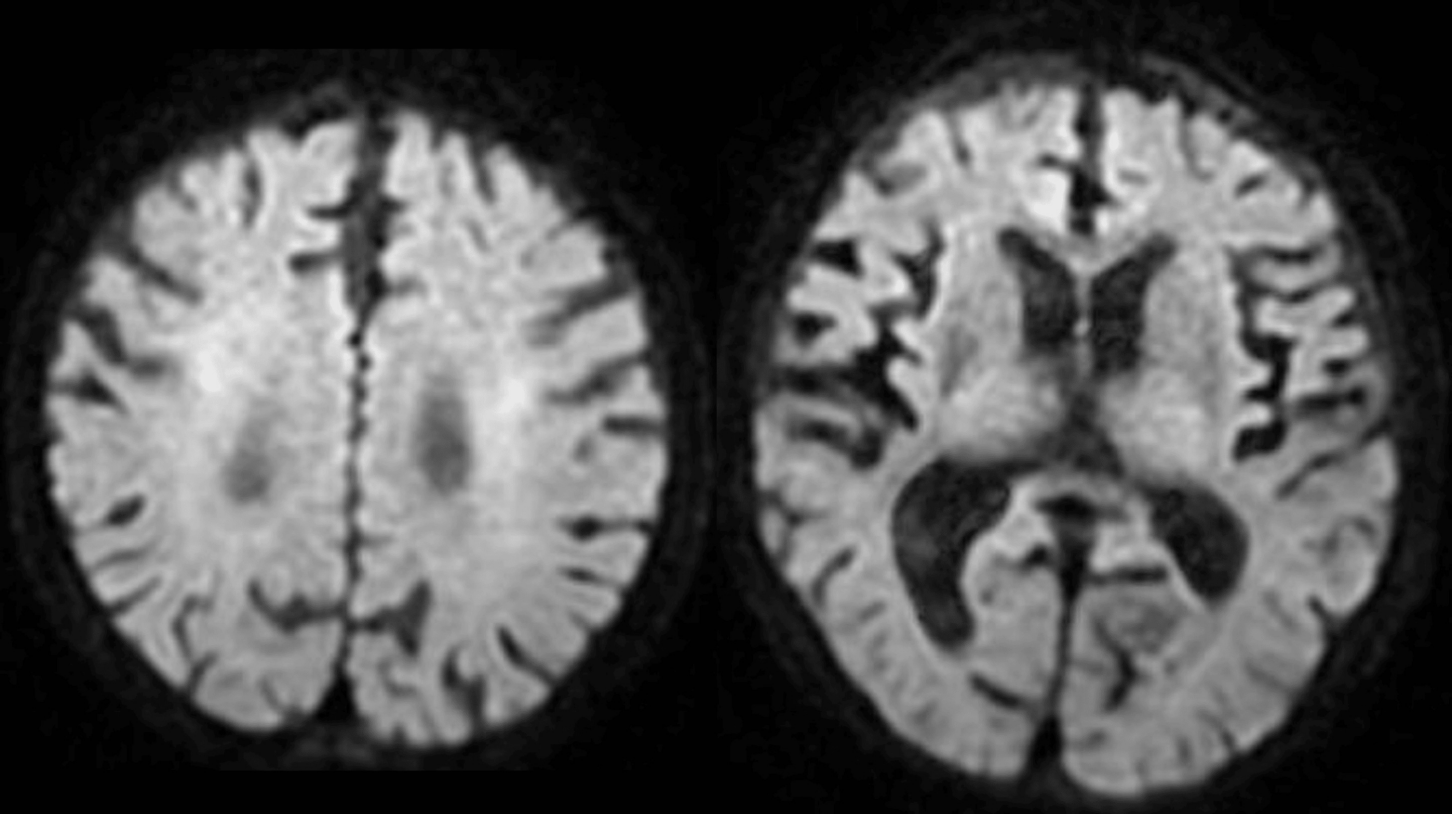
The results of diffusion-weighted brain magnetic resonance imaging (MRI) on day two of admission. Diffusion-weighted brain MRI showing attenuation of the areas of hyperintensities noted on admission.
